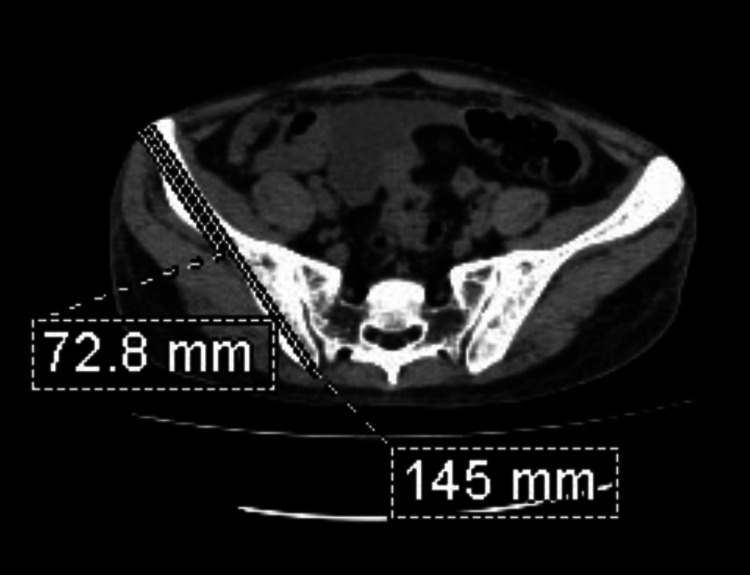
Axial CT section of the pelvis at the level of sacral ala with the midpoint of the line joining the anterior-superior iliac spine and the sacroiliac joint on the right side.
